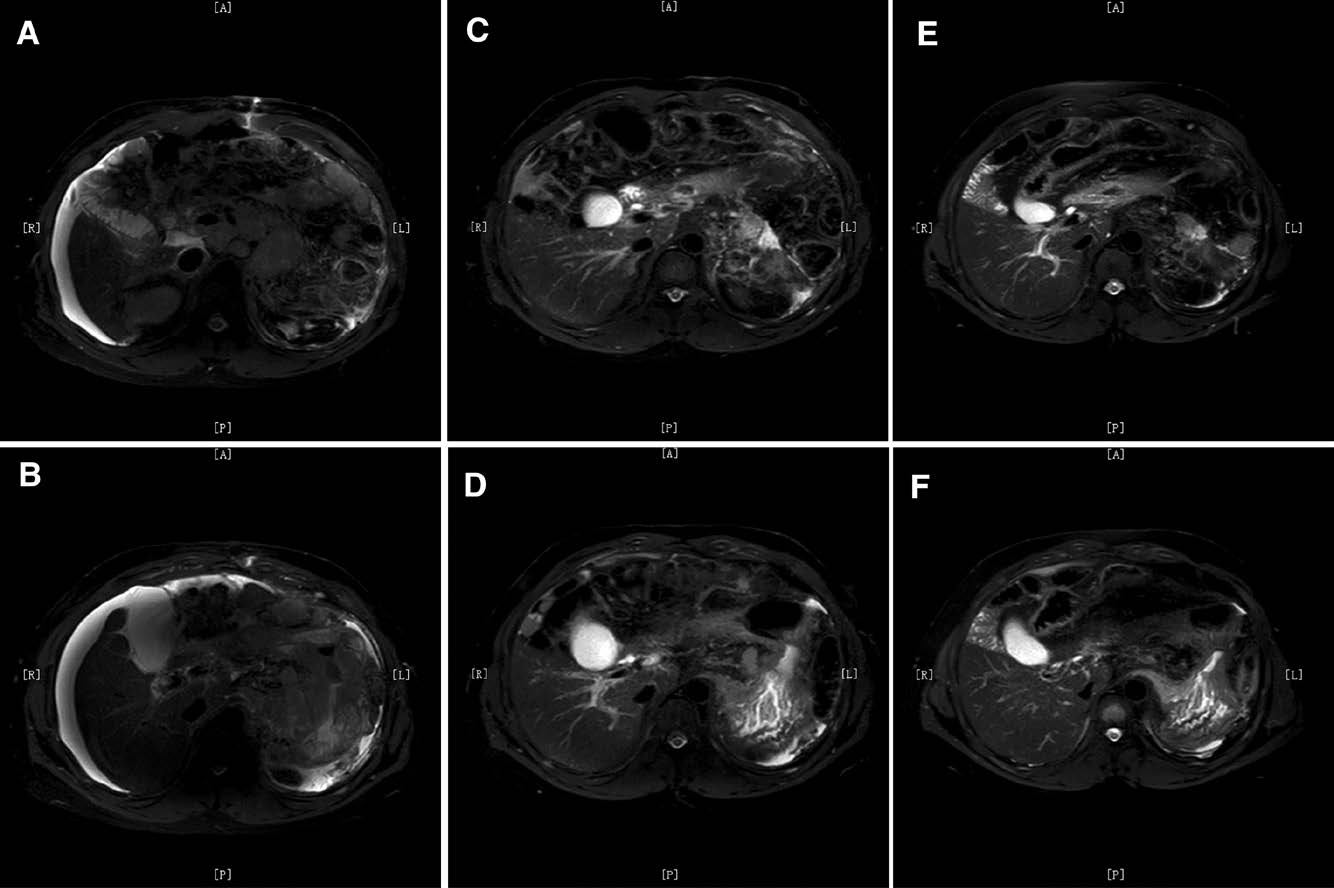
MRI examination before (A, B) and after (C, D) 7 months of ICI treatment showed reduction in the size of stomach wall lesions and lymph nodes, as well as resorption of abdominal fluid. There were no significant additional changes observed after 21 months of treatment (E, F).
